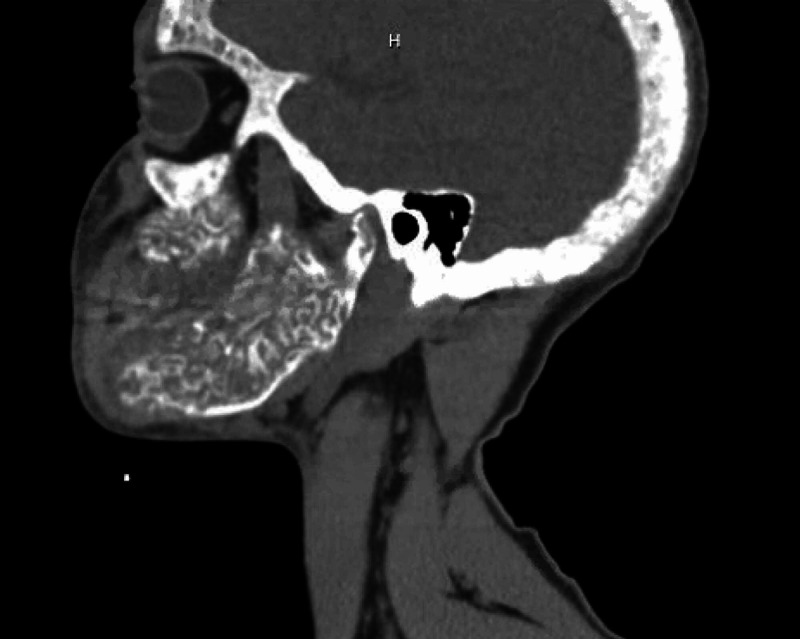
Sagittal view - Non-contrast CT showing markedly expanded mandible with prominent dense trabeculae. Calvarium appears abnormal, thickened with a smoking appearance.
